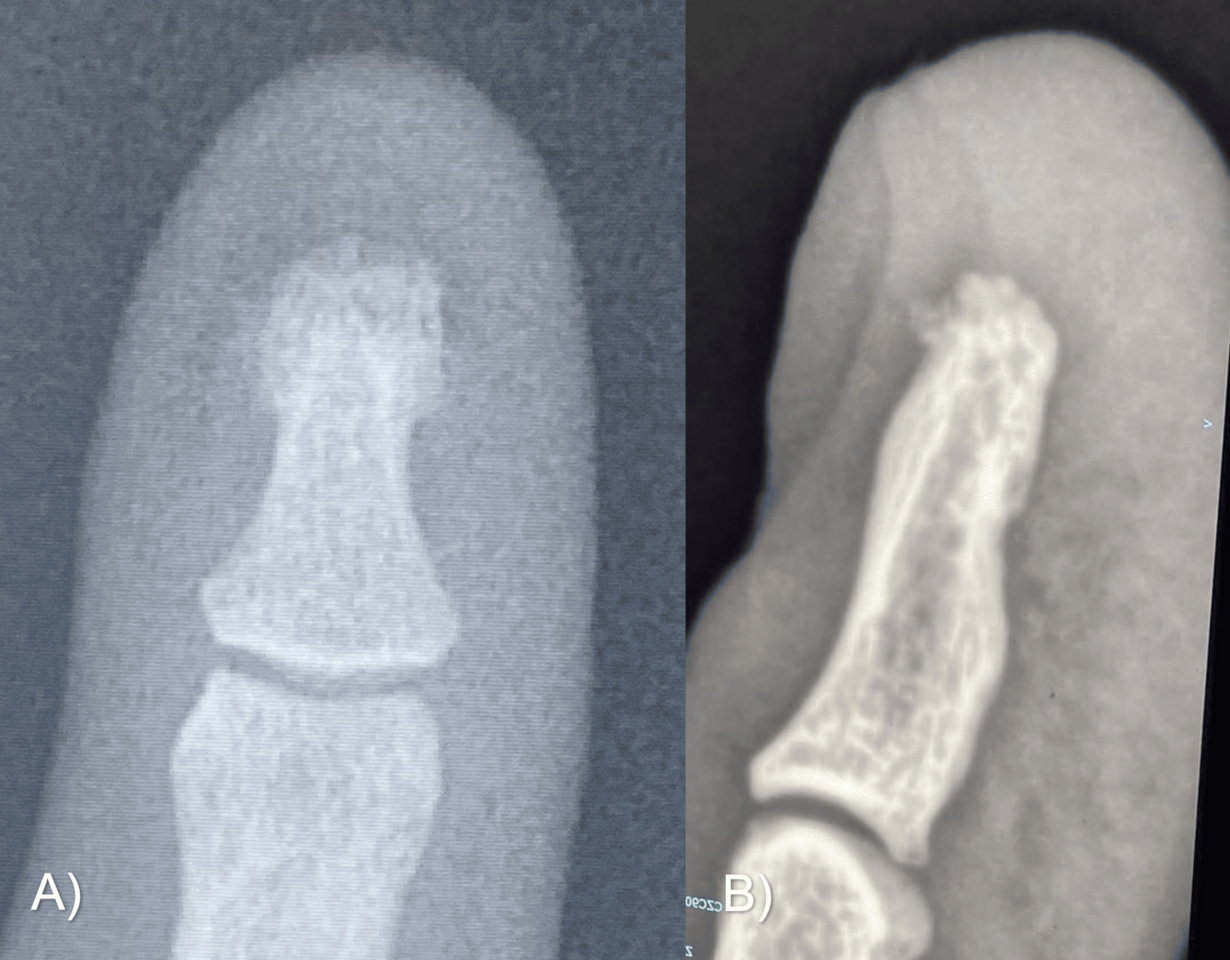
Radiographic examination in Case B Anteroposterior (A) and lateral (B) views of the left second digit with cortical lysis in the tuft of the distal phalanx
